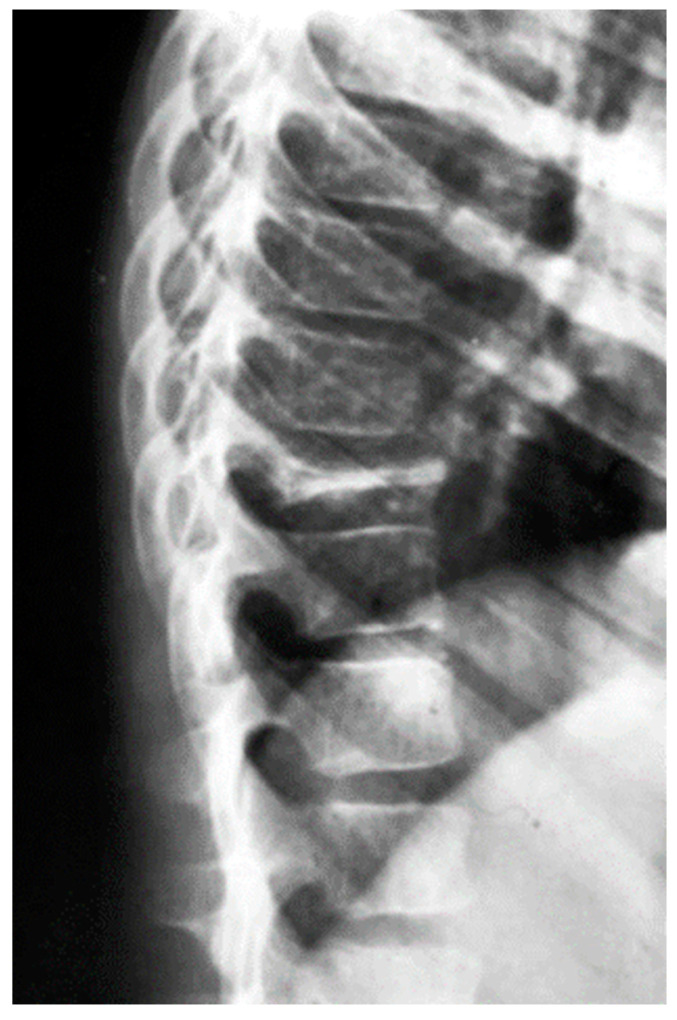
Lateral thoracic spine radiographs of a 7-year-old boy presenting with atraumatic back pain, with vertebra plana diagnosed as Langerhans cell histiocytosis (eosinophilic granuloma).
